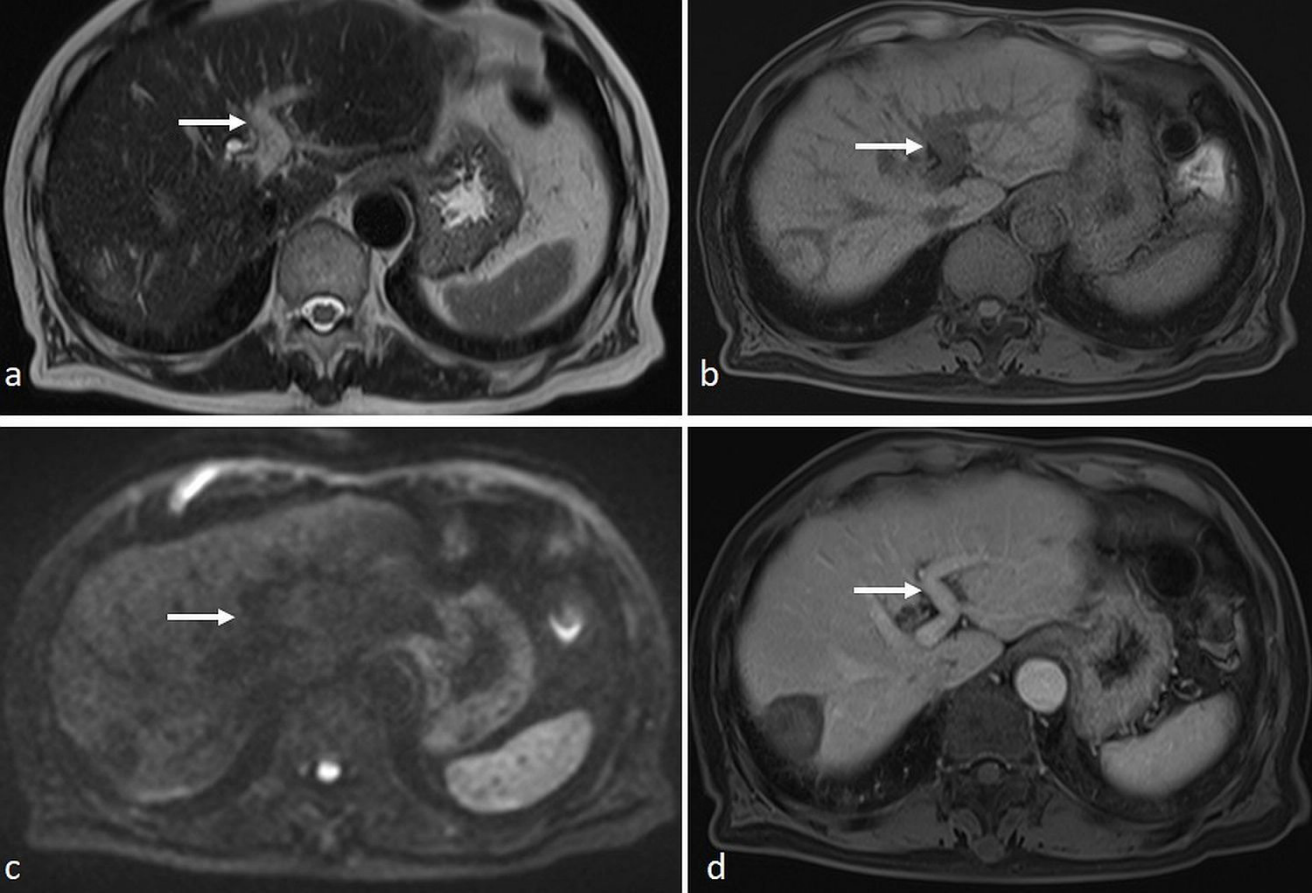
An 82-year-old gentleman with prior radiofrequency ablation of segment 7 HCC underwent MRI with Primovist. (a) On axial *T*
